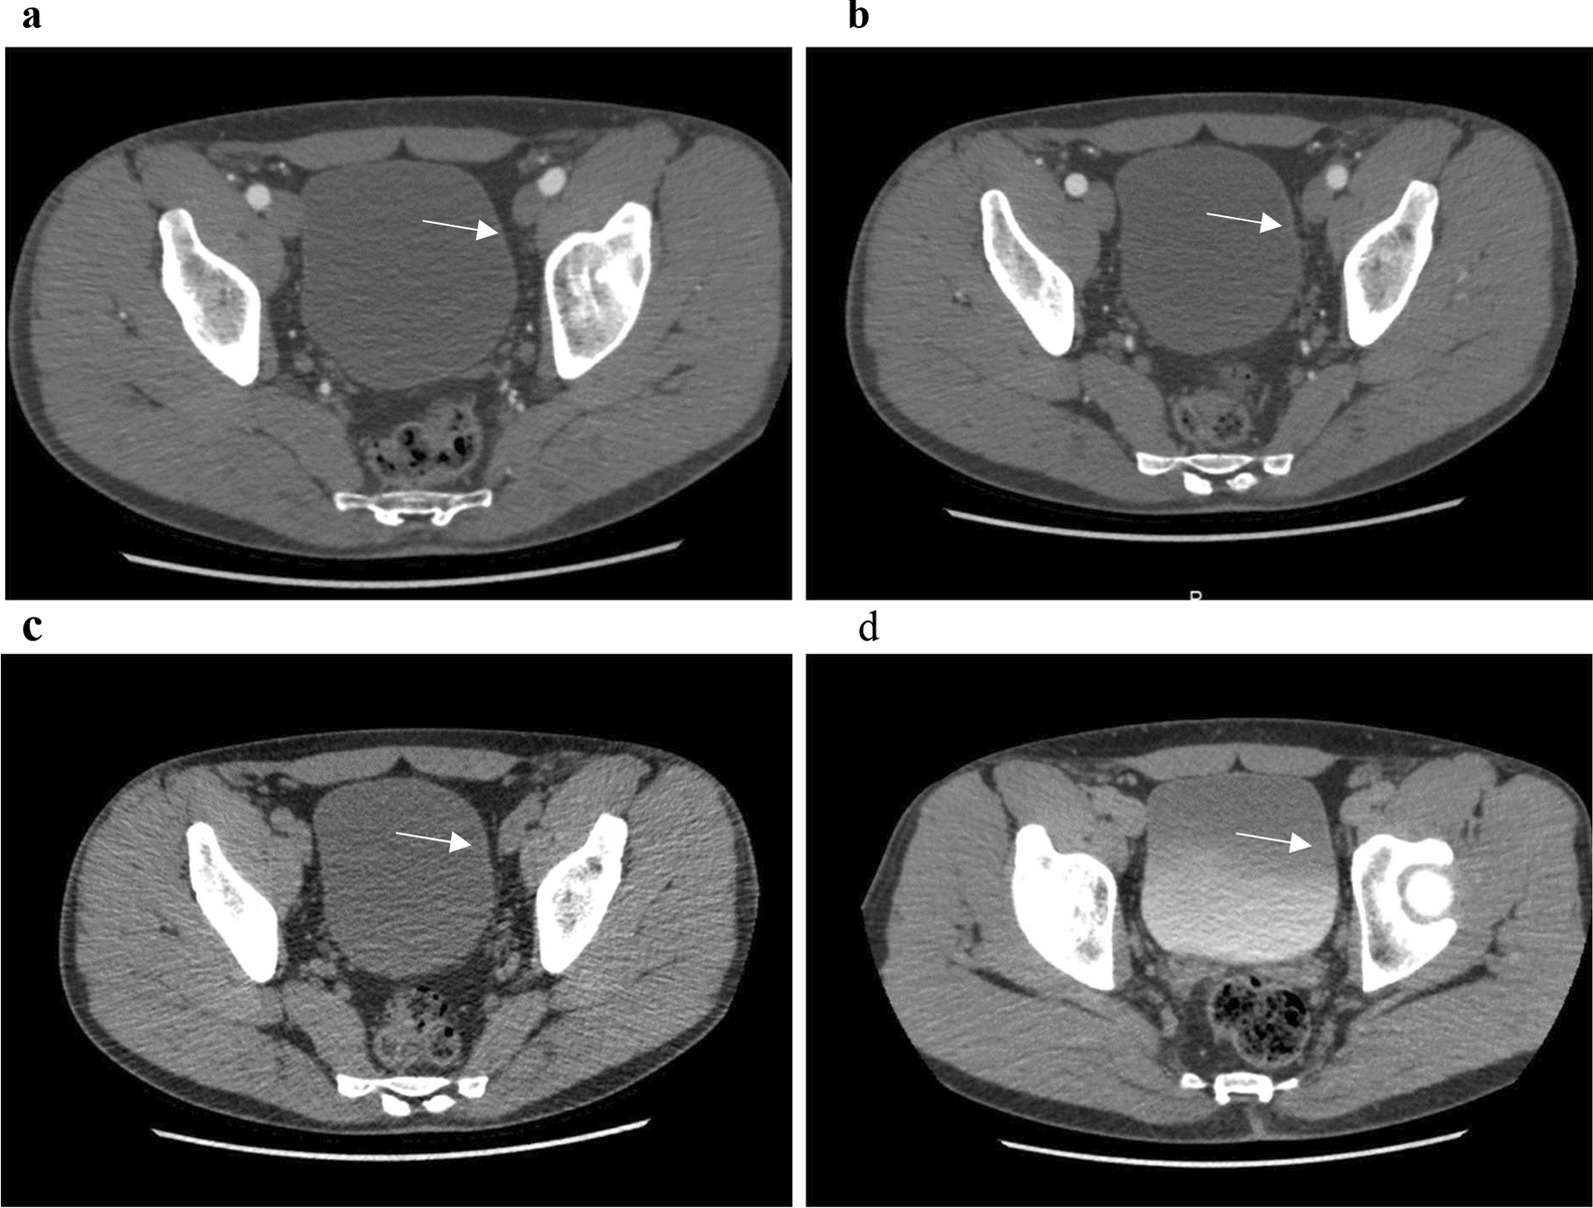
A flat (sessile) 3 cm T1G2 tumor in the urinary bladder which was missed in a computed tomography urography with high quality. An axial reconstruction in: a unenhanced phase, b corticomedullary phase (CMP), c nephrographic phase, and d excretory phase
